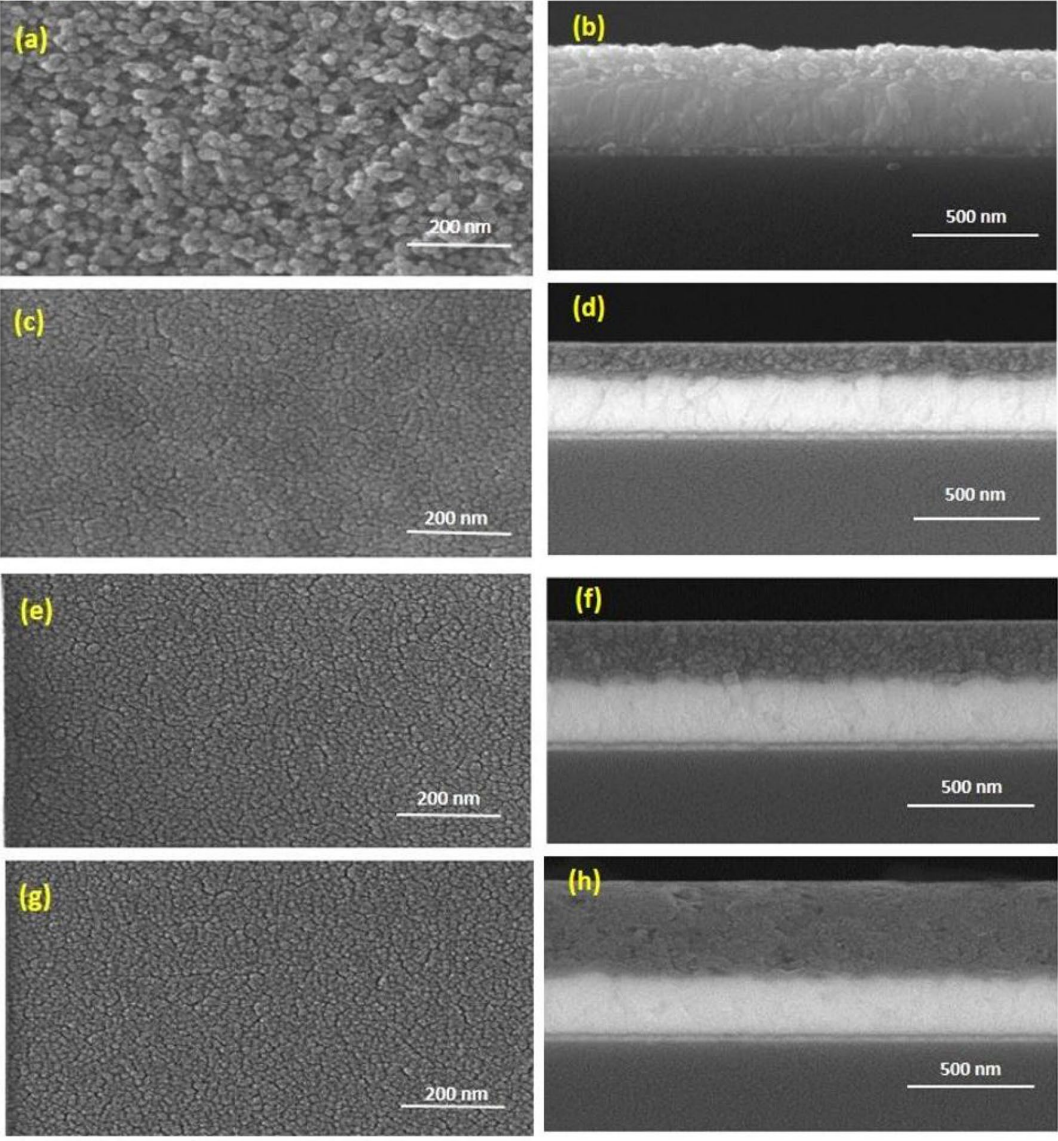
Top view and cross-sectional FE-SEM images of NC-TiO_2_ and CT-TiO_2_ layers on FTO coated glass (~ 15 Ω/sq) (a,b) RP-TiO_2_-1 layer, (c,d) CT-TiO_2_-1 layer, (e,f) CT-TiO_2_-2 layer, and (g,h) CT-TiO_2_-3 layer (4, 5, and 6 CT-TiO_2_ layers are shown in the supporting information).
